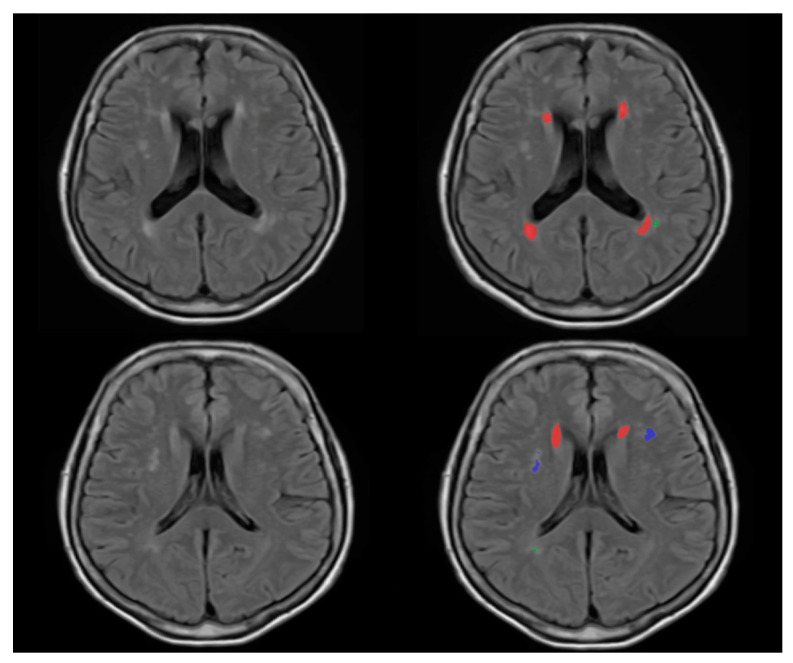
FLAIR MRI imaging of 65 years old female monozygotic twins (left column); the right side column pictures are the segmented version of the FLAIR images. The similarity of the anatomy and WMH of the cut is visible. According to our analysis, one twin has a total number of 17 WMHs (first row) and the other pair has a total number of 19 WMHs (second row). The red color represents the periventricular WMH and the blue and green colors represent the juxtacortical and deep white matter WMH—image from the Semmelweis University Medical Imaging Centre.
